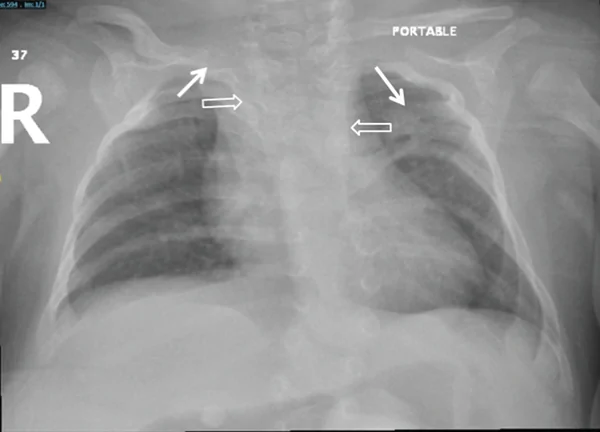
Anteroposterior view of the chest radiograph, demonstrating clear lungs without pleural effusion or pneumothorax and the presence of a normal cardiothoracic silhouette. Bilateral multilevel rib fusion anomalies (solid arrow) and congenital vertebral segmentation and fusion anomalies (empty arrow) are observed, contributing to scoliosis.
